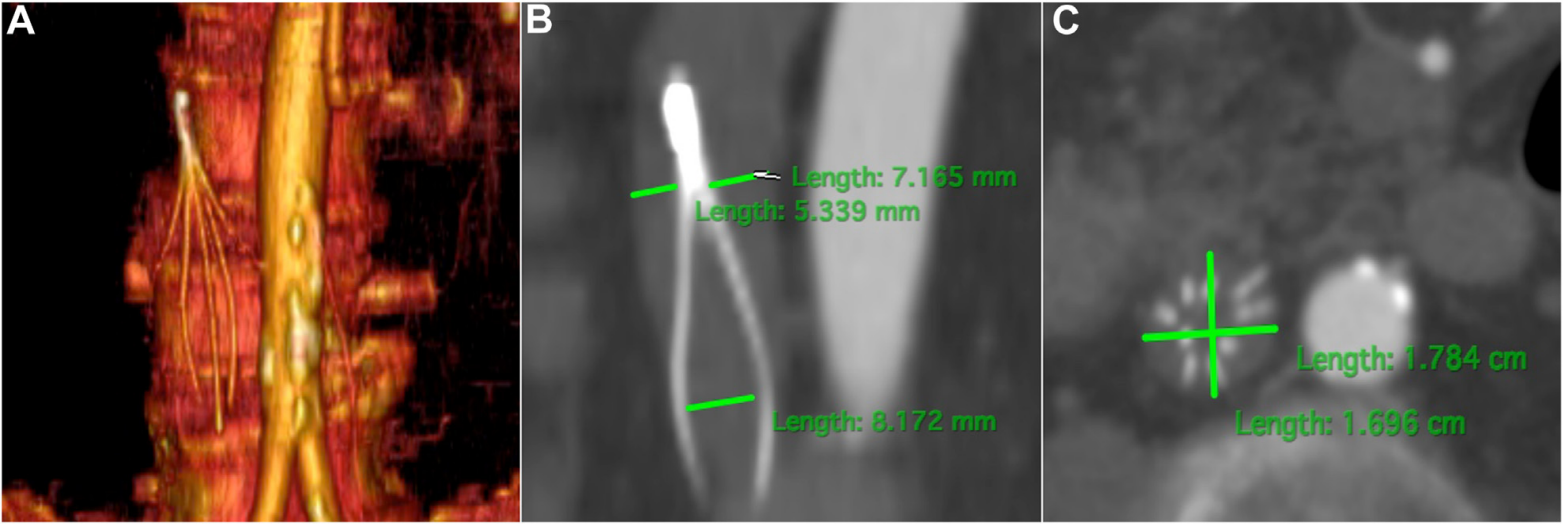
(A) 3-dimensional, (B) coronal, and (C) axial computed tomographic views of a filter implanted in the inferior vena cava.
